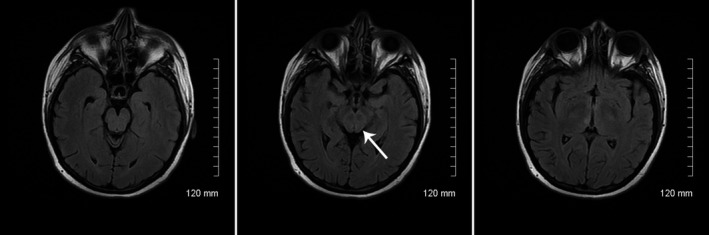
MRI on day 10. MRI T2‐weighted‐FLAIR (fluid‐attenuated inversion recovery) showed near complete resolution of the midbrain hyperintensity when compared to prior imaging (image in the center)
